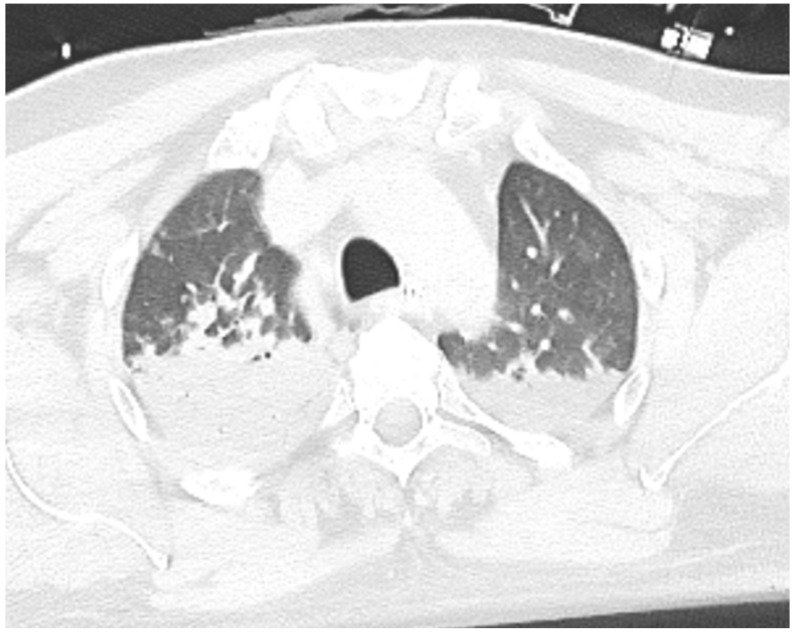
Chest CT scan on admission, showing bilateral diffuse consolidation areas, consistent with chemical pneumonitis.
